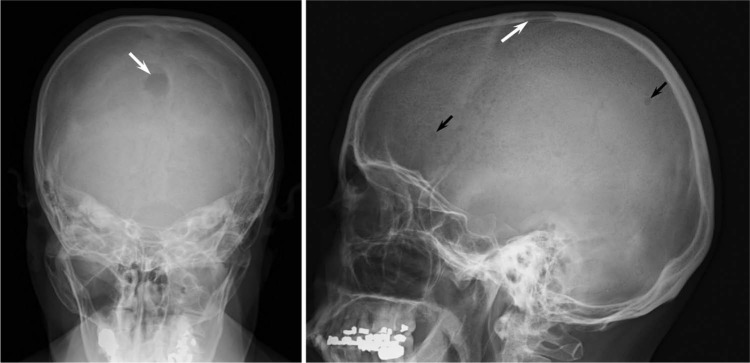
Skull radiographs. A well-defined 1.7-cm lytic lesion in the vertex of the skull was noted (white arrow) in addition to several other smaller lytic lesions (black arrows) in the calvarium suggestive for possible myelomatous involvement.
